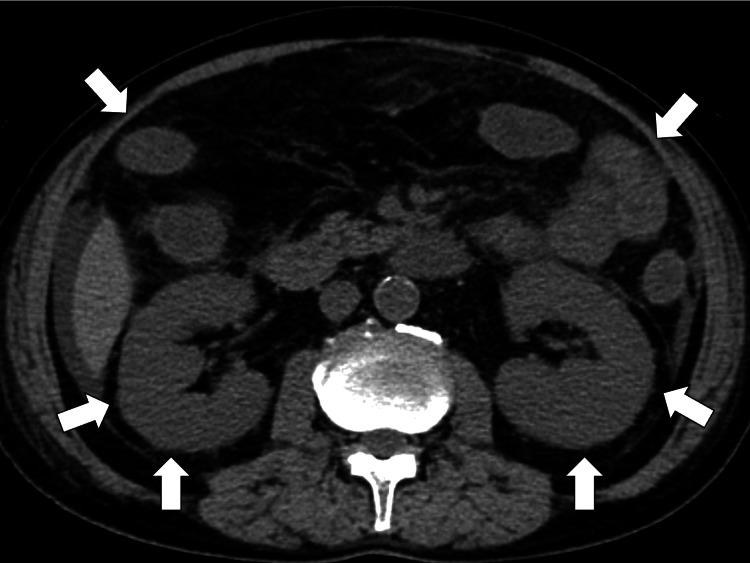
Abdominal CT showing the swelling of bilateral kidneys and diffuse edematous change of the small intestine and colon (white arrows) CT, computed tomography
